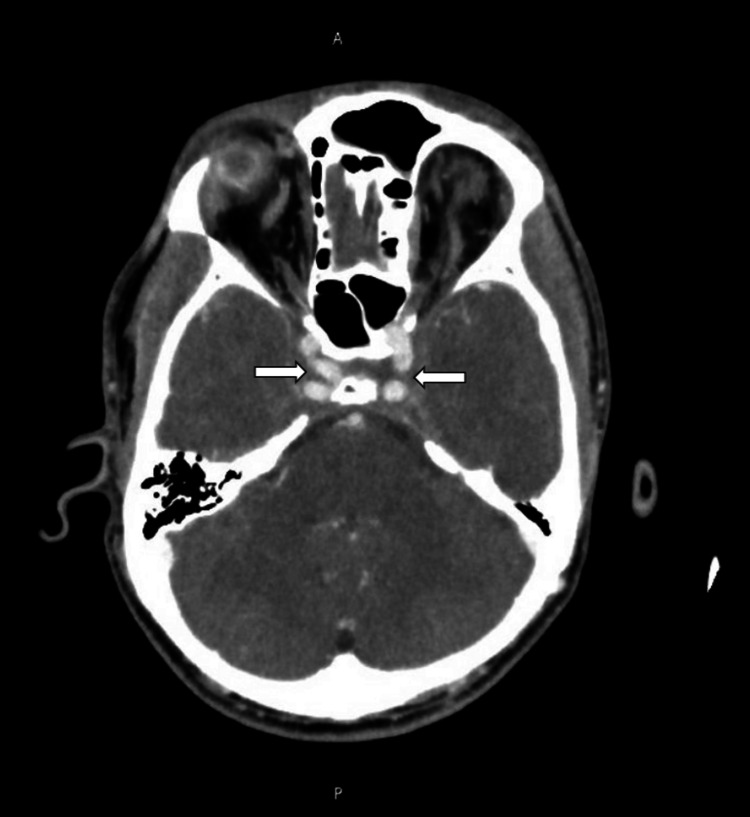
CT scan showing bilateral cavernous sinus thrombosis (white arrows)
